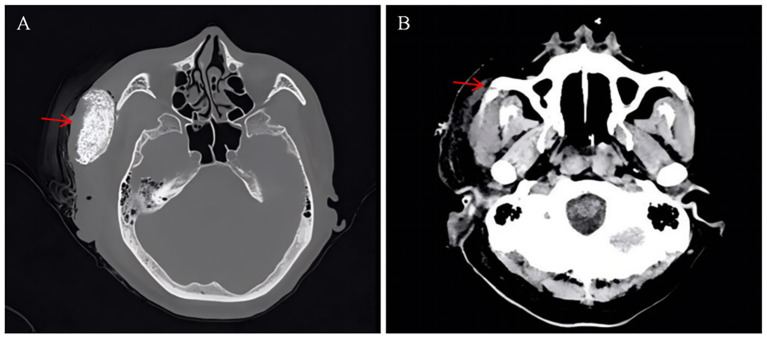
Maxillofacial computed tomography (CT) findings. (A) Axial CT image obtained after the initial surgical debridement. The red arrow indicates the extensive tissue defect which has been packed with radiopaque surgical gauze for hemostasis and drainage. (B) Axial CT image demonstrating the underlying facial trauma. The red arrow highlights the fracture of the right zygomatic bone, accompanied by significant adjacent soft tissue swelling.
